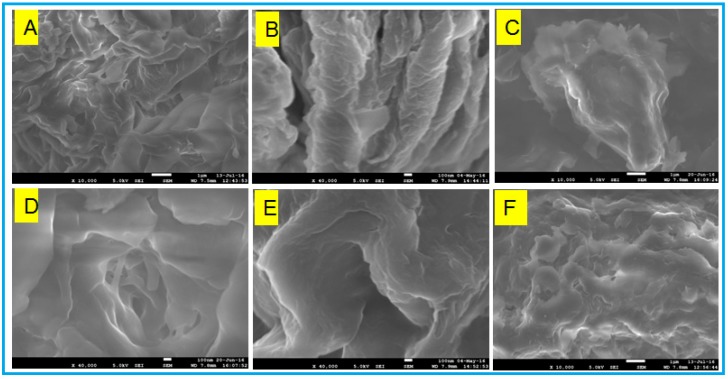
SEM images of xerogels of Gluconosemicarbazide gelator in DMSO/water mixture at a concentration of 30 mg/mL under different stimuli: (A) thermally-induced (1 µm); (B) SDS (100 nm); (C) KCl (1 μm); (D) NaCl (100 nm); (E) CaCl_2_ (100 nm); (F) ultrasound-induced (1 µm).
